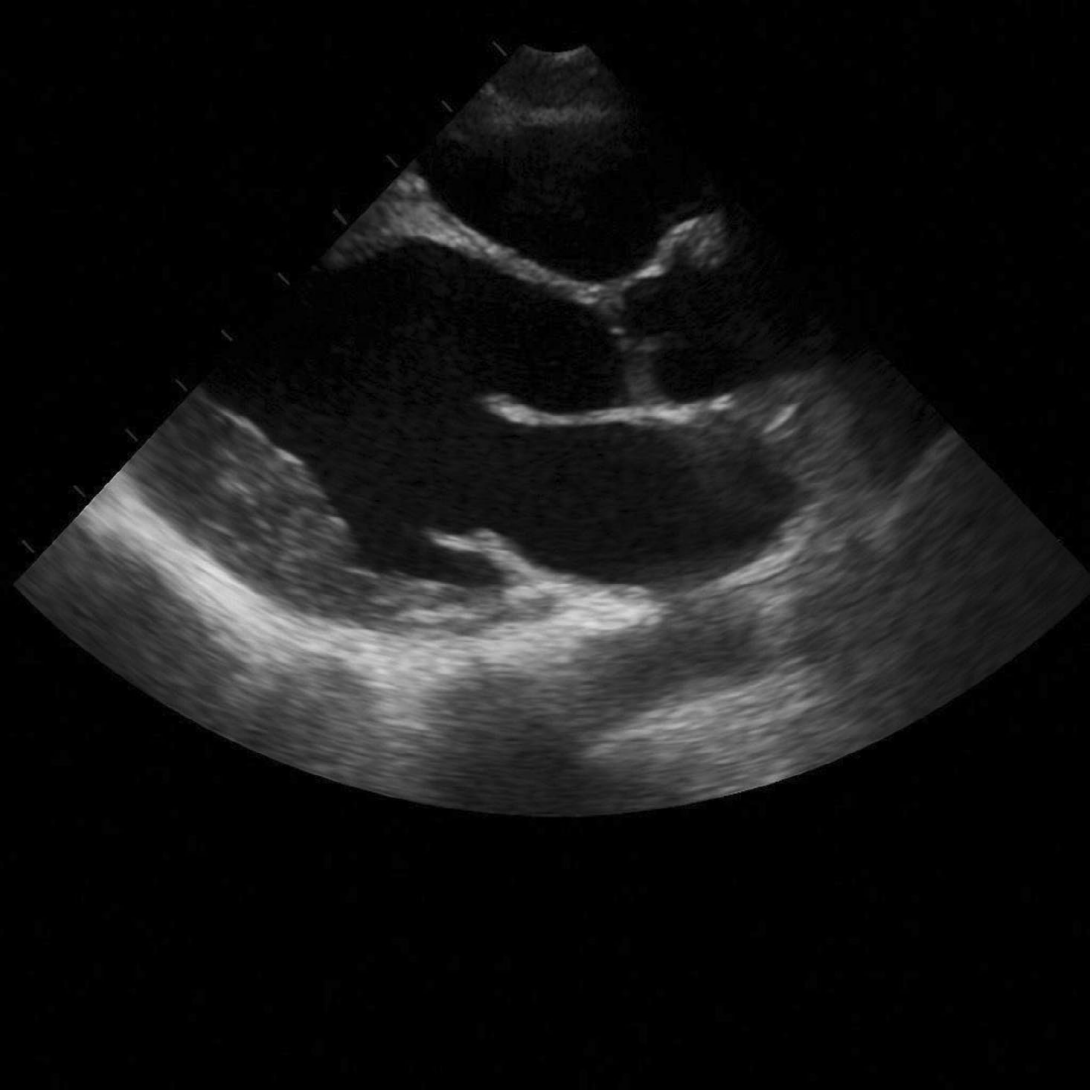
Echocardiogram of the patient showing normal left ventricular function without structural abnormalities, helping to differentiate hydroxychloroquine‐induced bradycardia from structural cardiac involvement of sarcoidosis.
